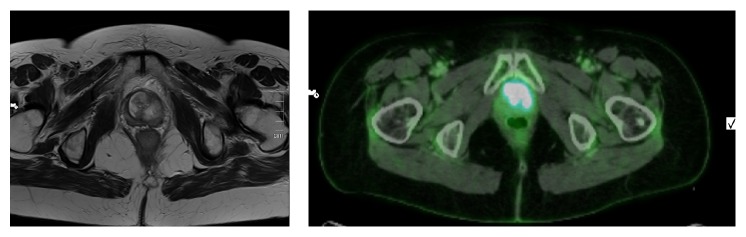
MRI and PET-CT scan visualizing the tumor.
